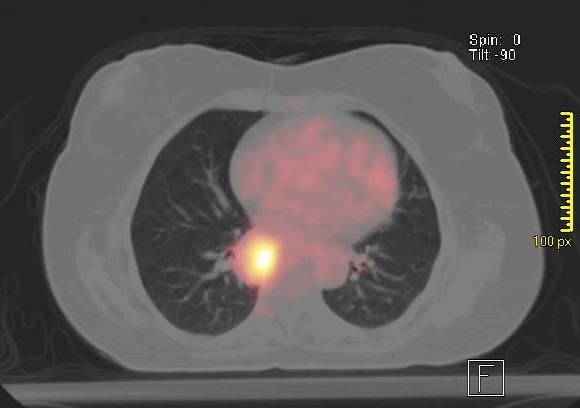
PET CT scan of chest showing increased uptake in nodules.
